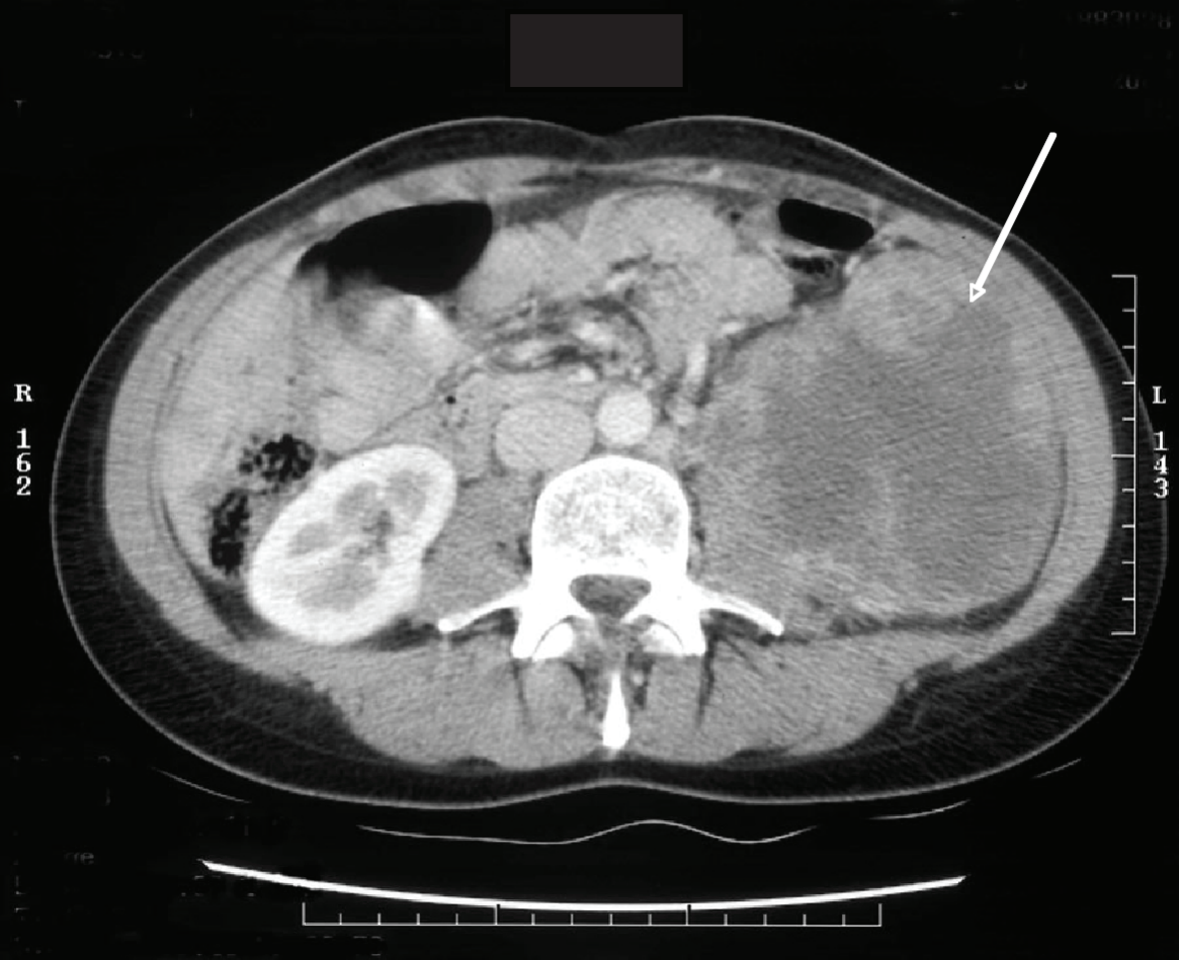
Post contrast CT demonstrating a 10 cm heterogeneously enhancing mass arising from the lower pole of the left kidney. It is centrally necrotic. The mass encroaches onto the left psoas and blocks the fat plane between itself and the left psoas muscle.
